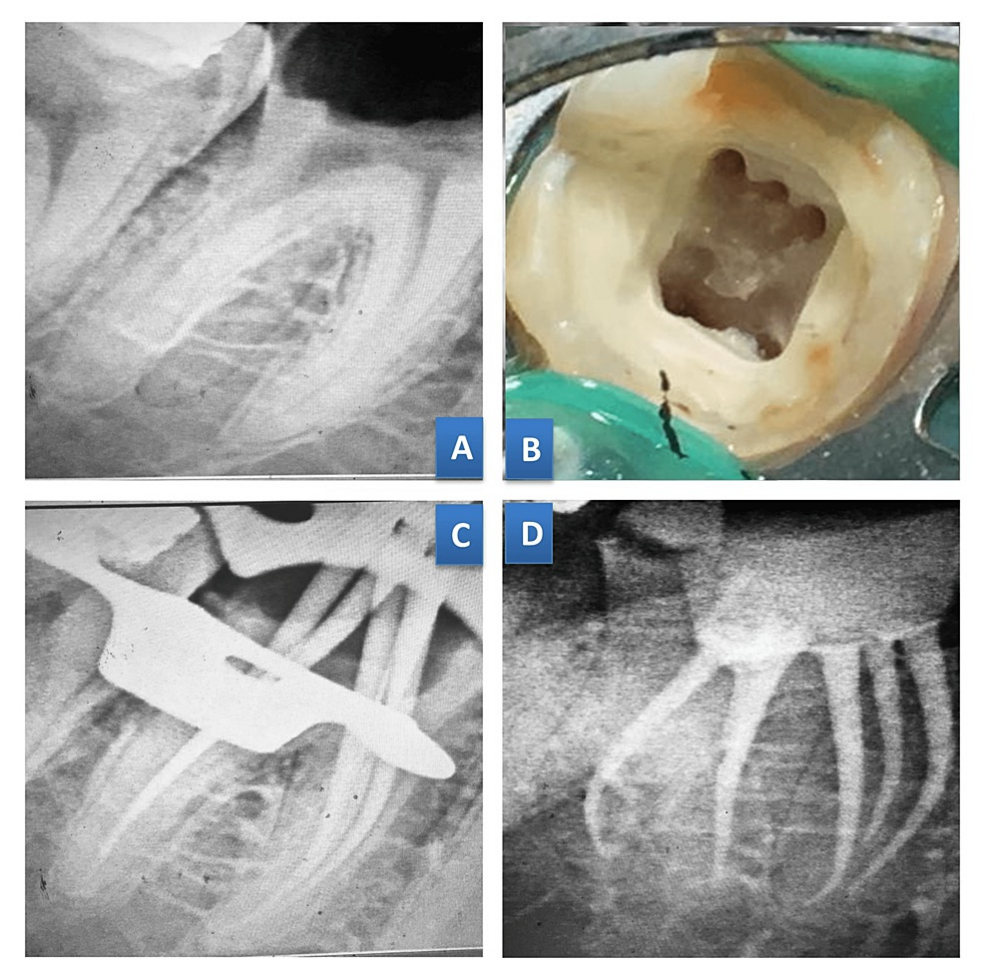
Case 3. Independent middle mesial canal with radix entomolaris 46. (A) Preoperative radiograph;  (B) Access cavity preparation; (C) Master cone radiograph; (D) Post-obturation radiograph
